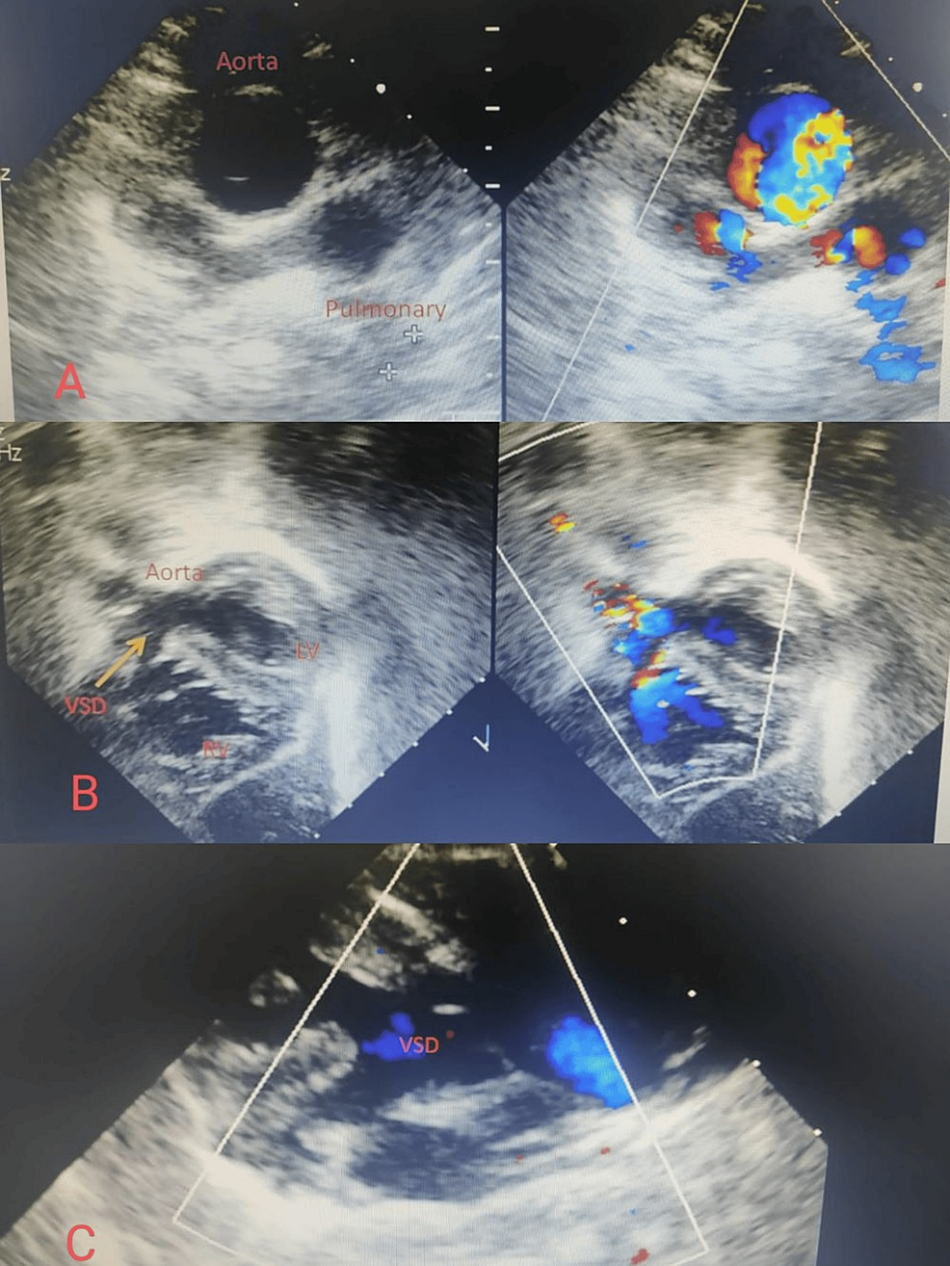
Two-dimensional echocardiography Image A: Showing great artery relationship with the aorta being anterior and to the right suggestive of transposition of great arteries, Image B: Subcostal view showing two good-sized ventricles and large VSDs and pulmonary outflow tract. Image C: Parasternal view showing large subpulmonic VSD with override VSD: Ventricular septal defect, LV: Left ventricle, RV: Right ventricle
